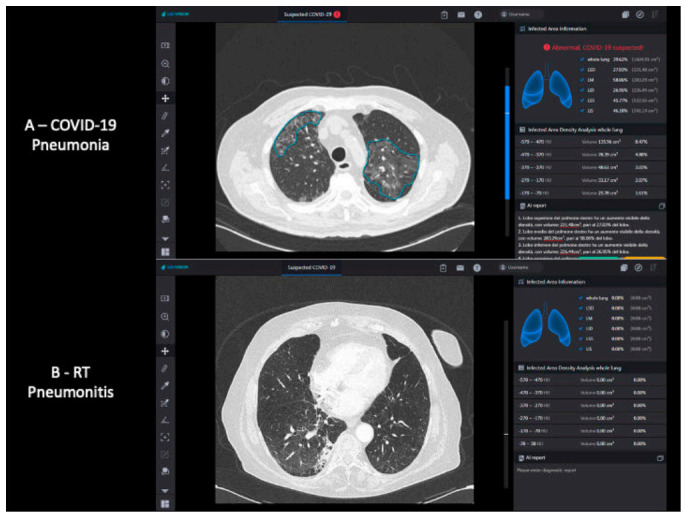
InferRead^TM^ CT Lung (COVID-19) system interface example; comparison between a patient with COVID-19 pneumonia (A) and a patient with RP (B). COVID-19 = coronavirus disease 2019; RP = radiation-therapy related pneumonitis.
